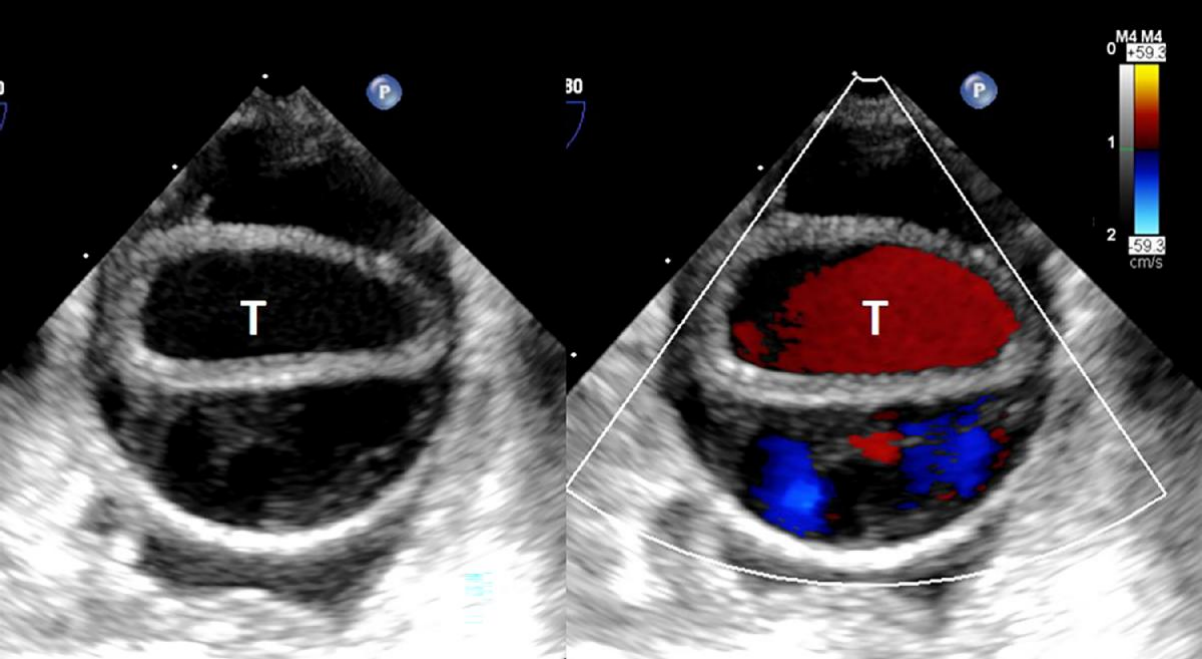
The type A aortic dissection. The aortic dissection extended to the descending aorta was revealed by transoesophageal echocardiogram with (right) and without (left) colour Doppler. The letter T indicates the true lumen of the affected vessel.
